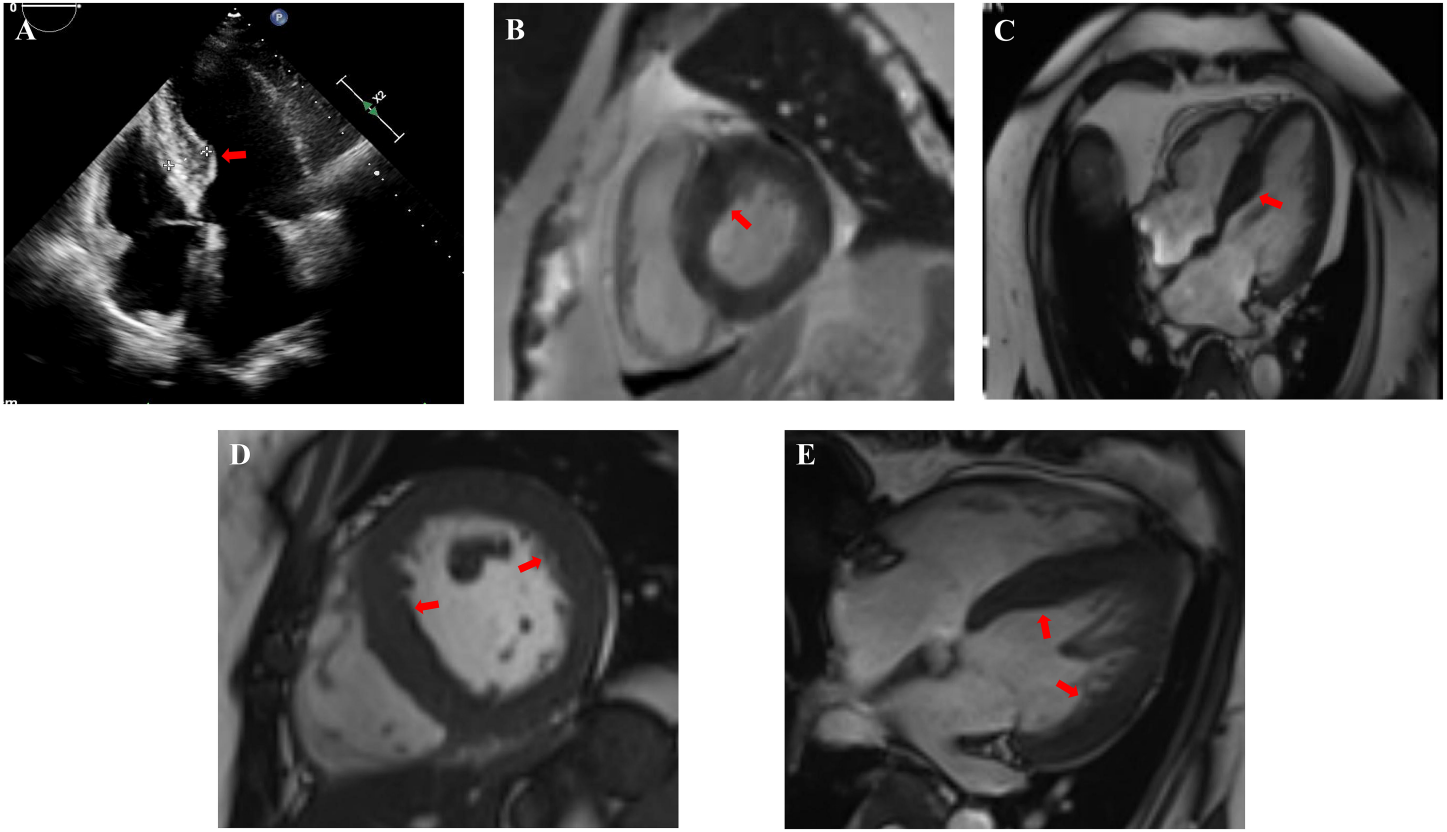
Cardiac images indicated non-uniformly thickened ventricular walls and hypertrophic cardiomyopathy of Case 2 (A–C) and Case 3 (D,E). (A) Echocardiogram in Case 2 showed significant thickening of the base of the interventricular septum. (B,C) Cardiac MRI in Case 2 showed the lateral wall of the left ventricle was about 8–10 mm and the thickening of the basal and intermediate segments of the interventricular septum was uneven with the thickest part about 20–22 mm (red arrow). (D,E) Cardiac MRI in Case 3 showed the lateral wall of the left ventricle was about 9–11 mm and the interventricular septum thickened, about 14–16 mm. The red arrows indicate non-uniformly thickened ventricular wall.
